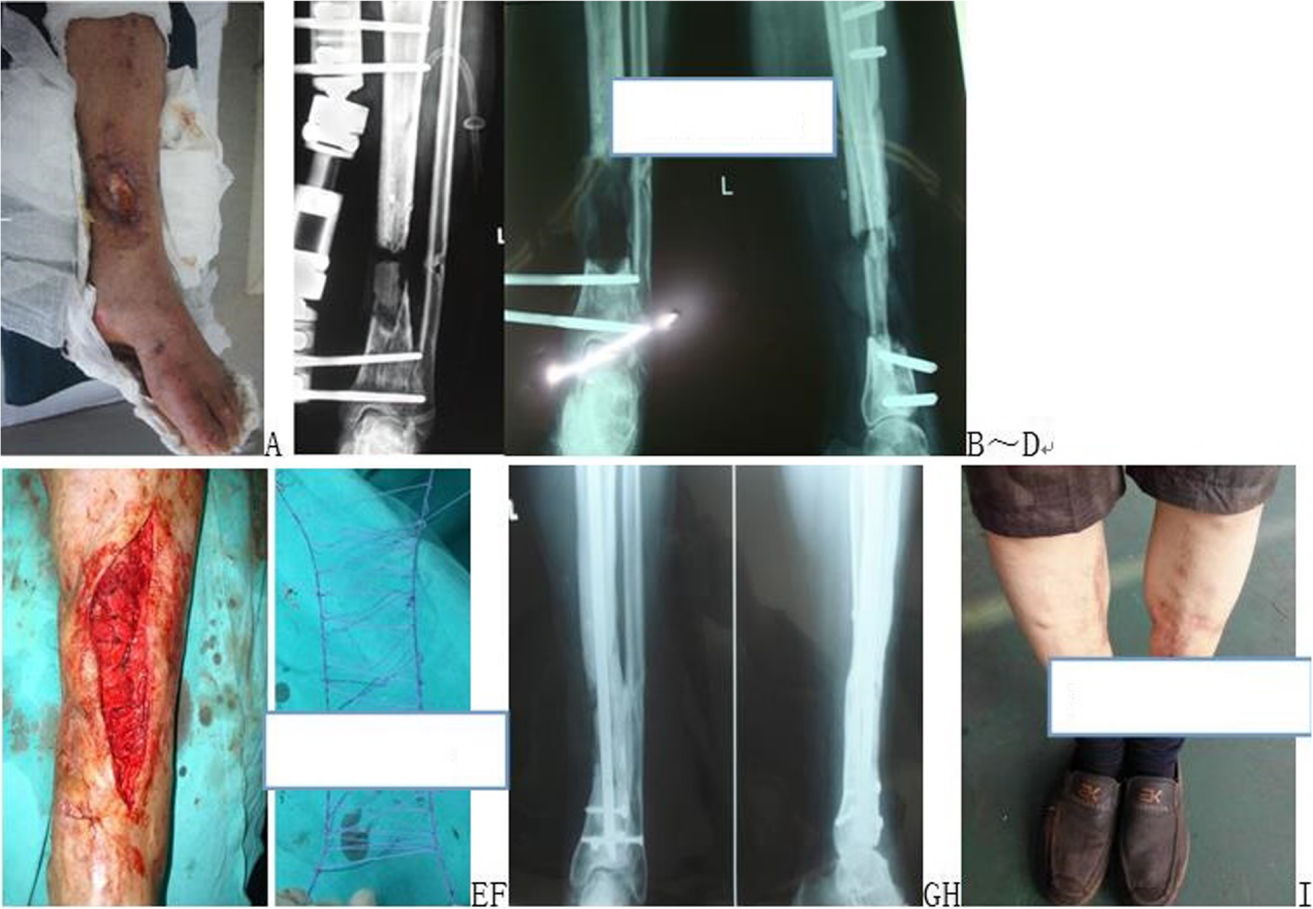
A patient with infected left tibial bone defects treated with line mesh wrapped cancellous bone graft in second intention surgery. a Appearance of the bone defects. b-d Preoperative X-ray films. e, f Surgical procedure using the line mesh material and the wrapped cancellous bone graft technique. g, h Appearance 1 year postsurgery. i Appearance of bone healing one year after bone grafting
